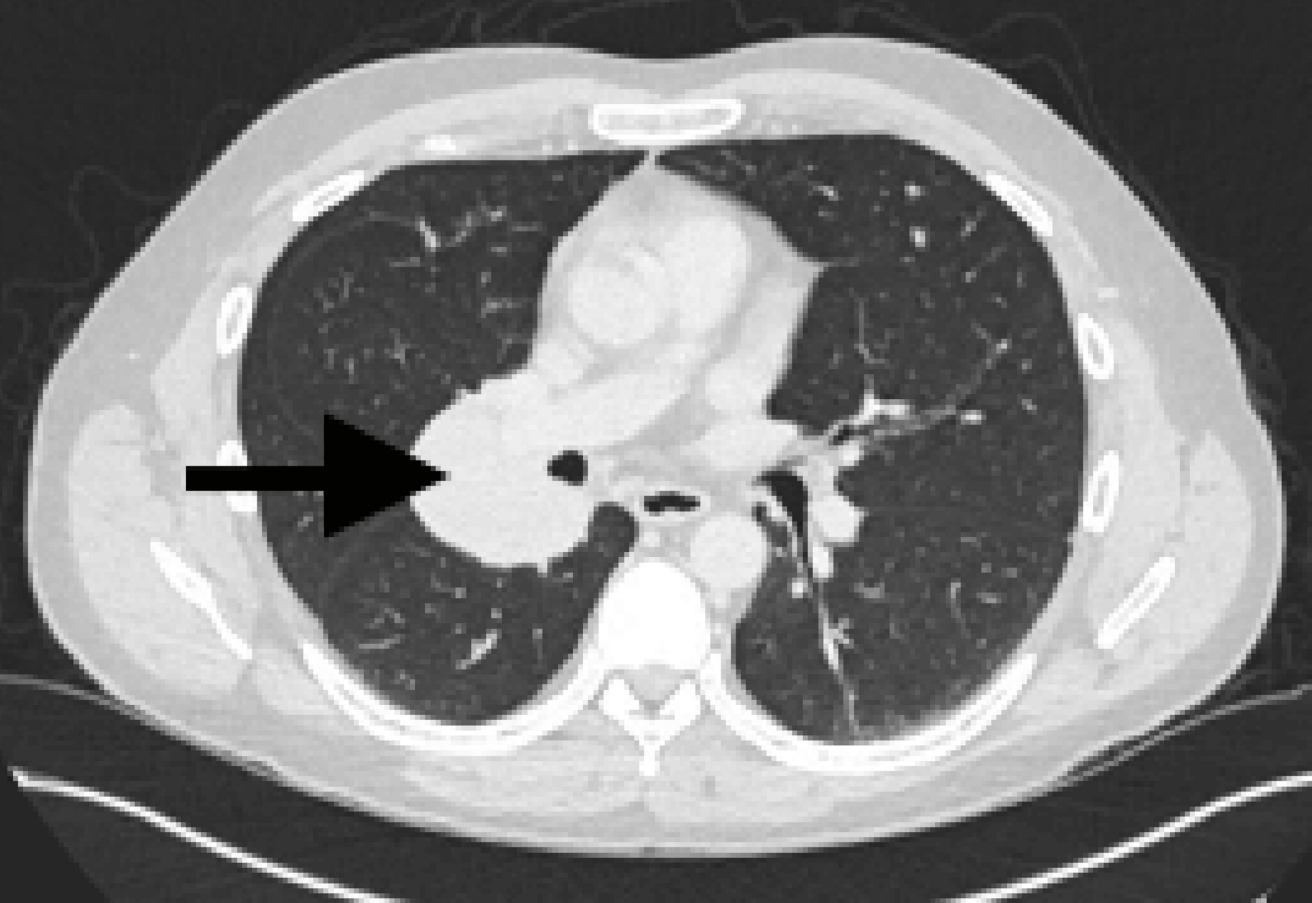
Computerised tomography (CT) in axial view showing a well-defined 6.4-cm right hilar mass (black arrow).
